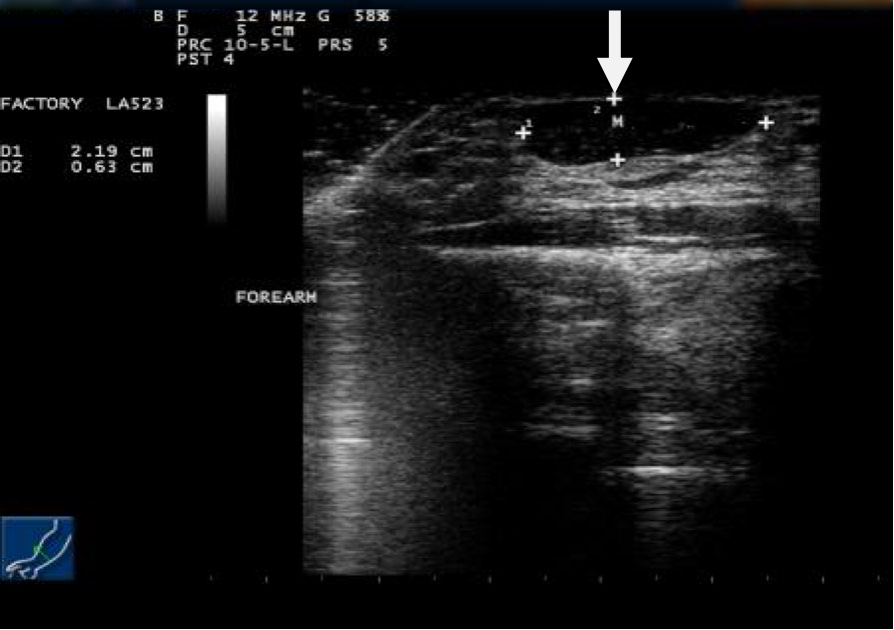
Ultrasonographic image of the right forearm in transverse view revealing the muscle mass (the *arrow* indicates the hypertrophied muscle)
